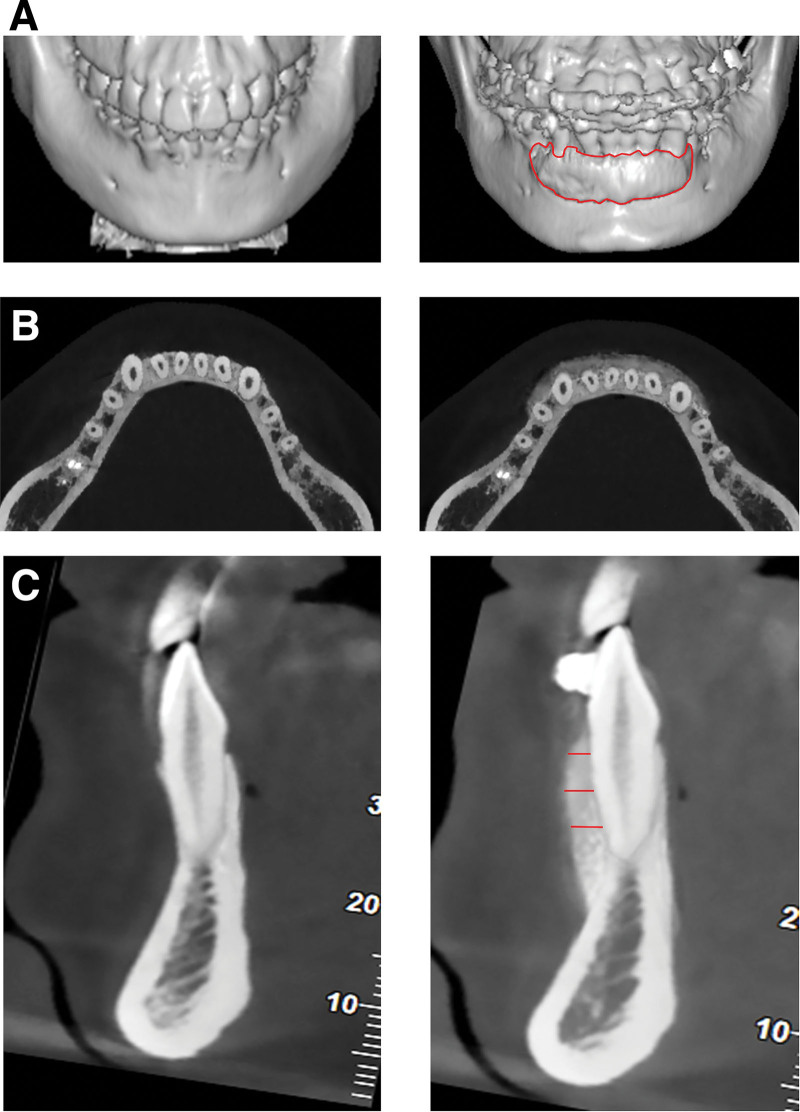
Preoperative and postoperative CBCT images. (A) Image-based 3-dimensional reconstruction before surgery and at postoperative 3-month; area of bone augmentation was colored by red; (B) CBCT images of horizontal view shows significant alveolar bone augmentation in the same mandibular anterior area at postoperative 3-month; (C) images of buccal-lingual view shows significant alveolar bone augmentation in the buccal radicular surface after PAOO; red lines show BTRC1/3, BTRM1/3 and BTRA1/3. BTRA1/3 = bone thickness of root apical 1/3, BTRC1/3 = bone thickness of root coronal 1/3, BTRM1/3 = bone thickness of root middle 1/3, CBCT = cone-beam computerized tomography, PAOO = periodontally accelerated osteogenic orthodontics.
